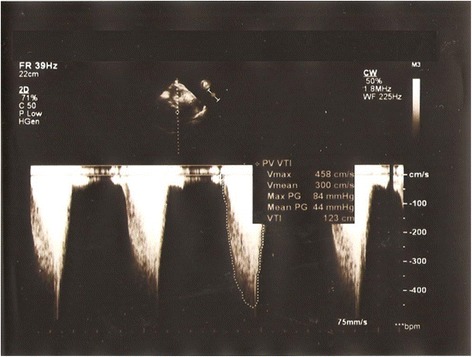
Continuous wave Doppler of the main pulmonary artery indicating a mean pressure gradient of 44 mmHg at the site of compression
